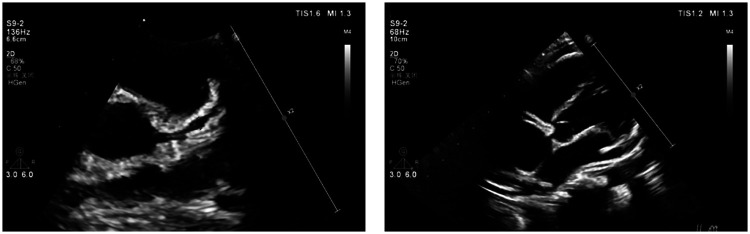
Echocardiography at six-month follow-up after discharge.
